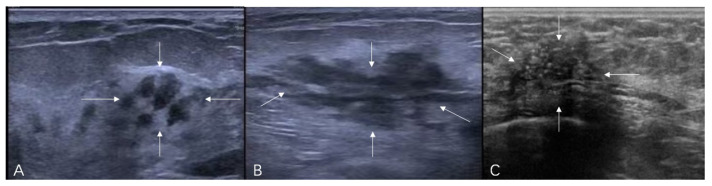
Ultrasound features of non-mass breast lesions of Type I. (A) A case of ductal carcinoma in situ (DCIS). Ultrasound shows patchy hypoechoic areas in the breast (indicated by the white arrows); (B) a case of DCIS. Ultrasound reveals mottled hypoechoic areas within the breast (indicated by the white arrows); (C) a case of DCIS. Ultrasound demonstrates ill-defined hypoechoic areas in the breast (indicated by the white arrows).
